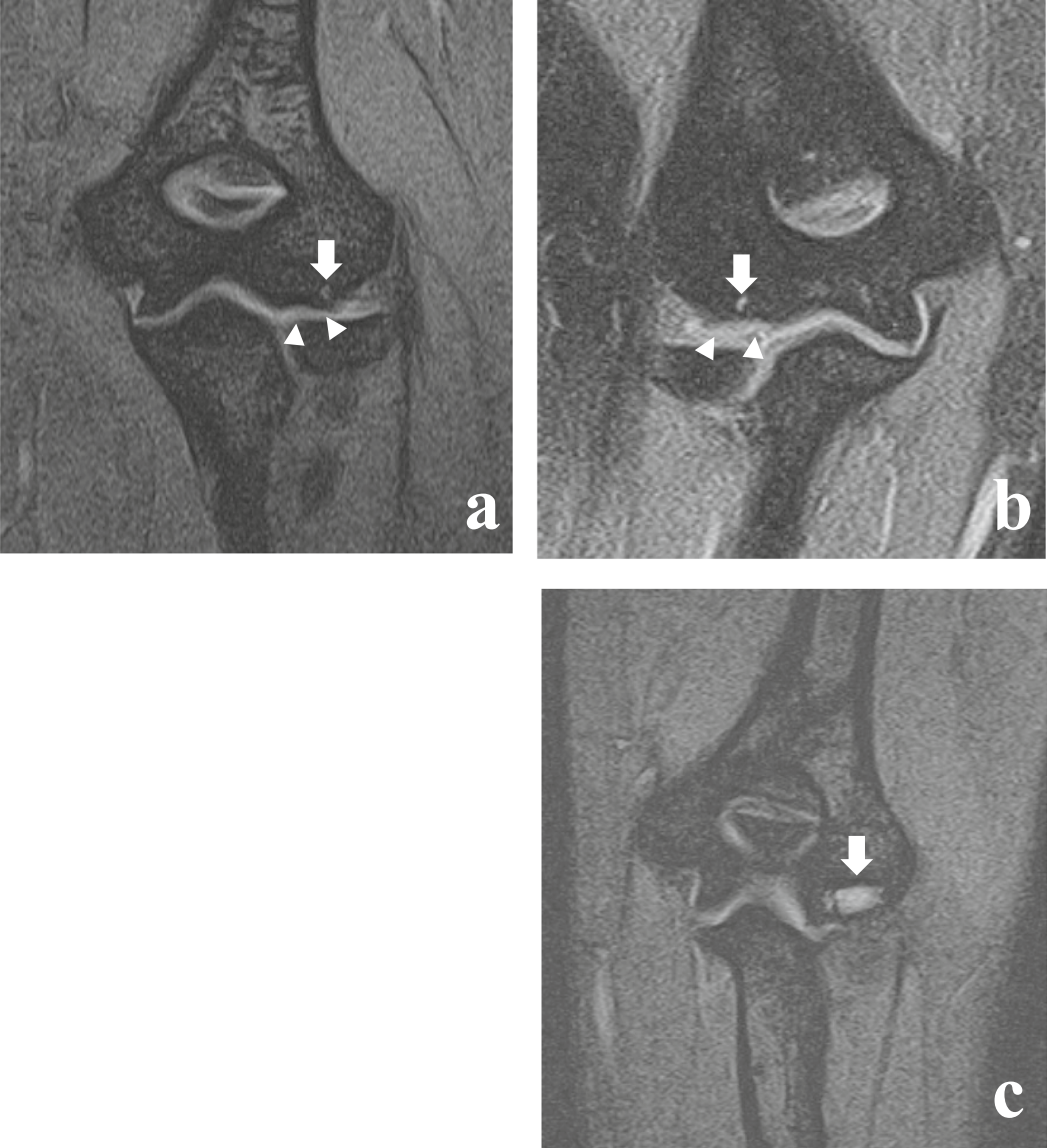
MRI finding of various LP lesions. a, b A 32-year-old woman with bilateral LP lesions, which appeared as a small cyst at the LP part of the capitulum (arrows) in the left (a) and right (b). The arrowheads suggest that cartilage is observed, and cysts exist in the subchondral bone. These findings suggest that the entity of this cyst is apparently different from the subchondral cysts observed in osteoarthritis. c A 27-year-old woman with a LP lesion, which appears as a large, flat-shaped cyst with a septum (arrow) at the LP part of the capitulum. Image was acquired by T2* weighted imaging
